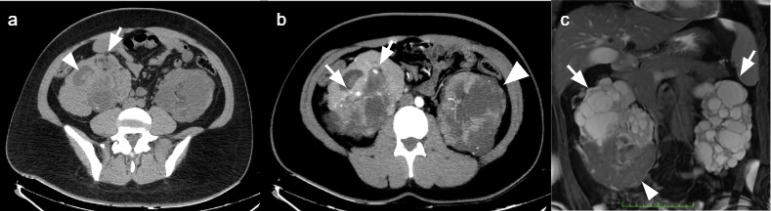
a) Non-enhanced CT evidencing multiple small masses with fat attenuation in the right kidney lower pole consistent with AMLs (arrow). Hyperattenuating material filling the dilated renal pelvis and calyces due to hematuria (arrowhead). b) Maximum intensity projection arterial phase CT evidencing pseudo-aneurysms/intrarenal aneurysms in the right kidney lower pole (arrows) and bilateral cortical cysts (arrowhead). c) Coronal T2-weighted fat-suppressed magnetic resonance image shows multiple bilateral simple cysts with thin regular walls (arrows). Renal parenchyma is shown in the lower pole of the right kidney (arrowhead).
